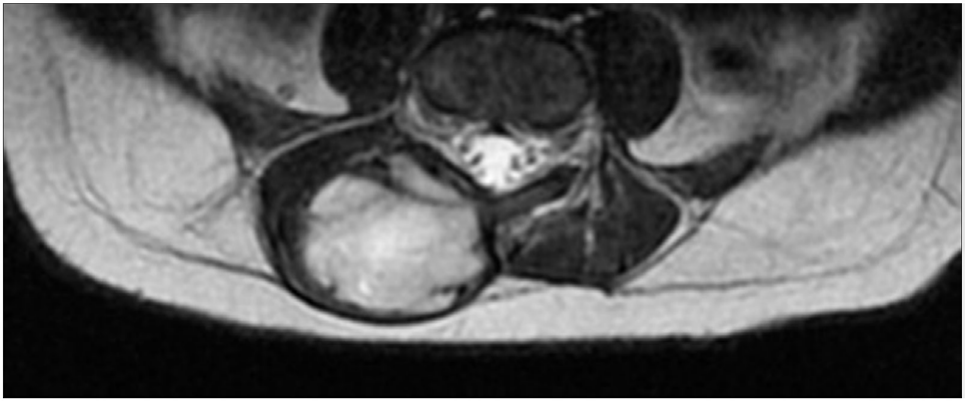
MRI before surgical excision (axial).
